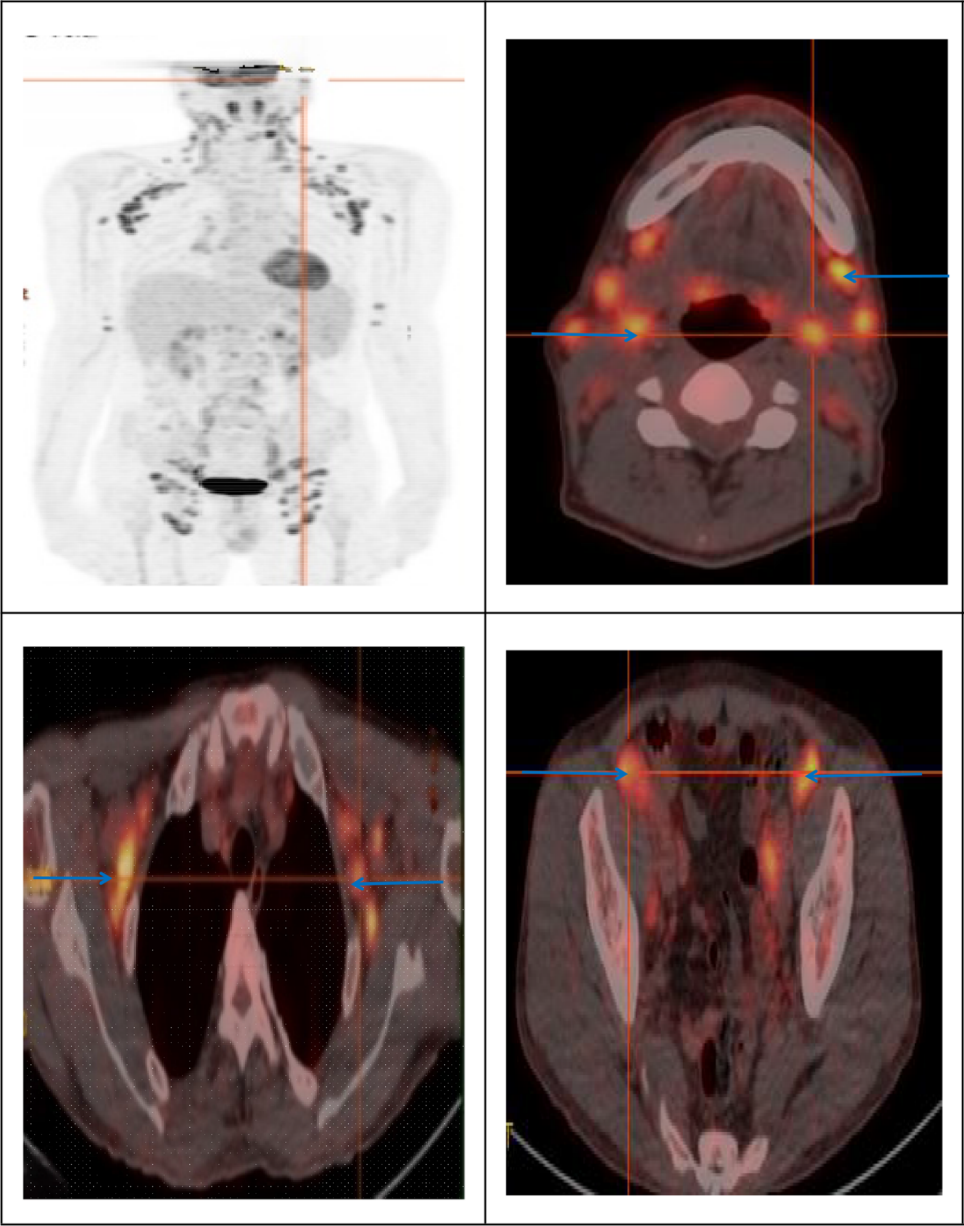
Before treatment, the patient’s pretreatment 18F-FDG PET imaging showed multiple sites of high uptake.
